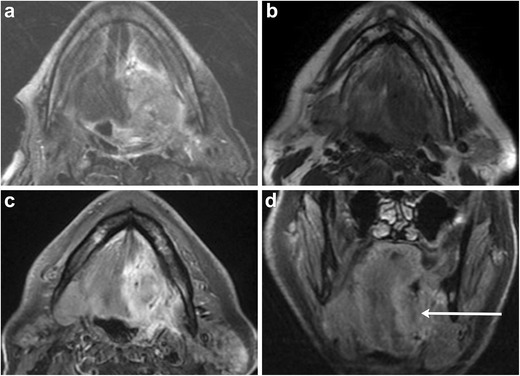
Squamous cell carcinoma involving the left sublingual space. Infiltrative lesion centred within the left sublingual space, which demonstrates heterogenous intermediate to high signal on T2-weighted MRI (a) and isointensity compared with adjacent musculature on T1-weighted MRI (b). c Post-contrast axial and d coronal T1-weighted MR images reveal heterogenous enhancement (*arrow*)
